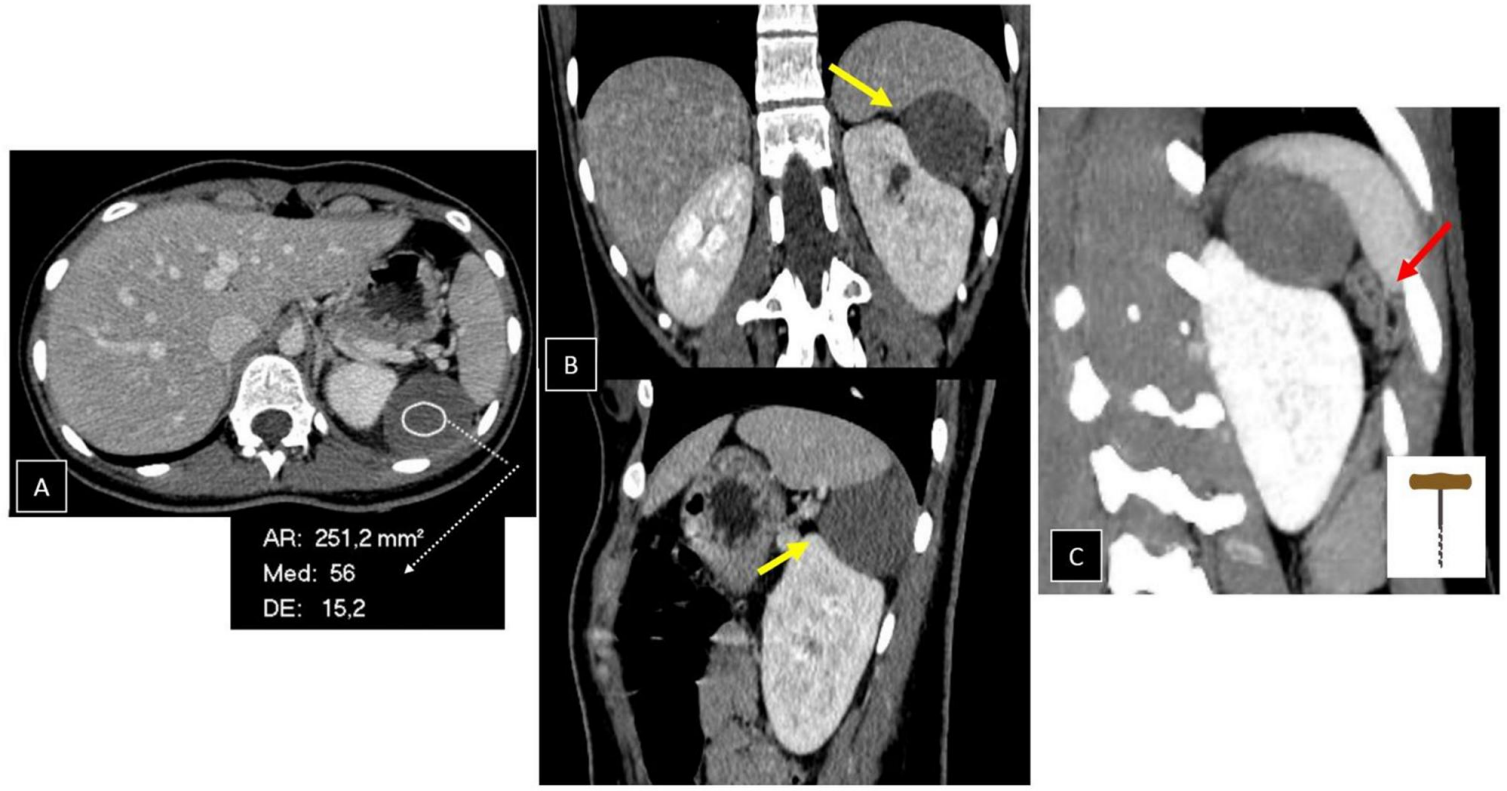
A contrast-enhanced abdominal CT in the venous phase shows a well-defined, likely unilocular lesion measuring 43 × 46 mm, located under the spleen, with a density range of 40-60 Hounsfield units. (A) The lesion mildly indents the lower border of the spleen and the upper-lateral aspect of the left kidney (yellow arrows), without clear organ dependence (B). On the right (C), an oblique coronal section is shown, where the tortuous dilation of the left gastroepiploic vessels of the twisted pedicle is visible, resembling a corkscrew shape (red arrow). No indications of subacute haemorrhage are observed.
